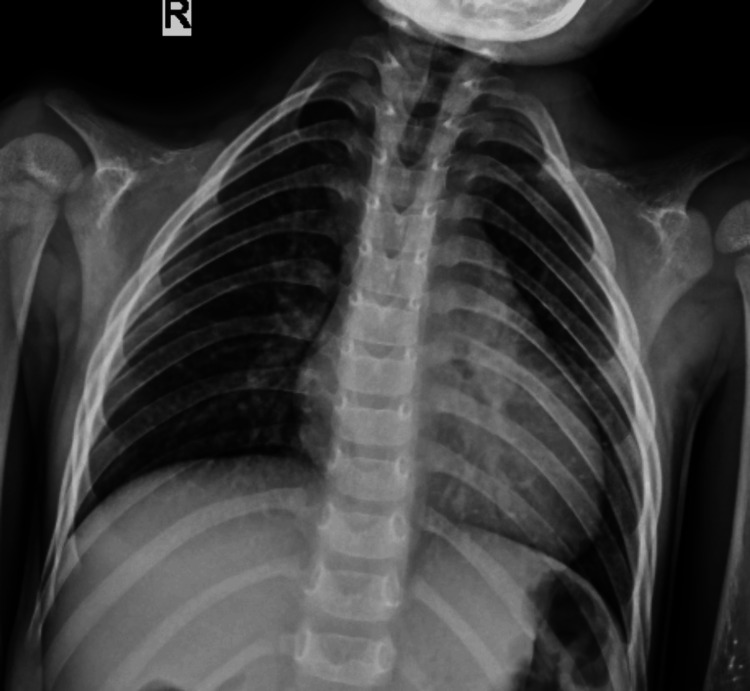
Frontal chest radiograph of this seven-year-old girl showing bilateral absence of clavicles and a bell-shaped thoracic cage.
